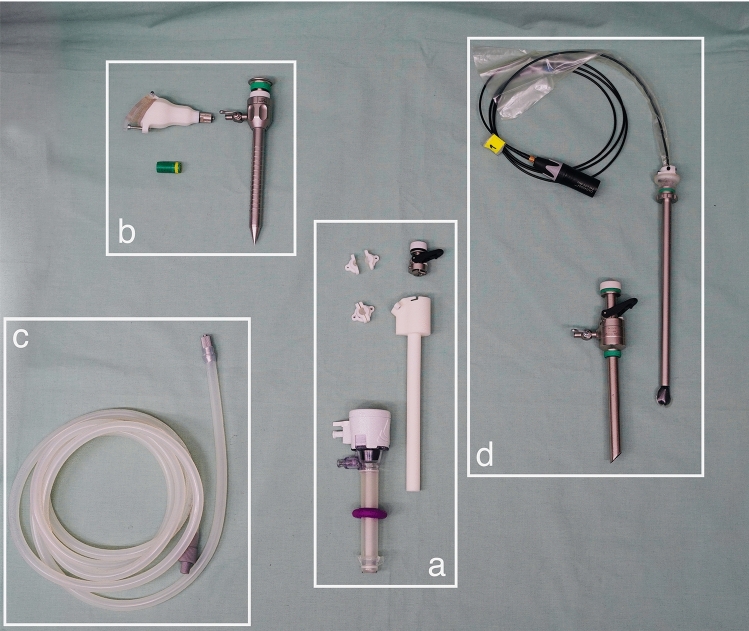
Overview of different prototypes for evaluation: a dual-channel insert, b Luer-Lock adapter, c silicon tube, d reference microphone covered by ultrasound probe sleeve
